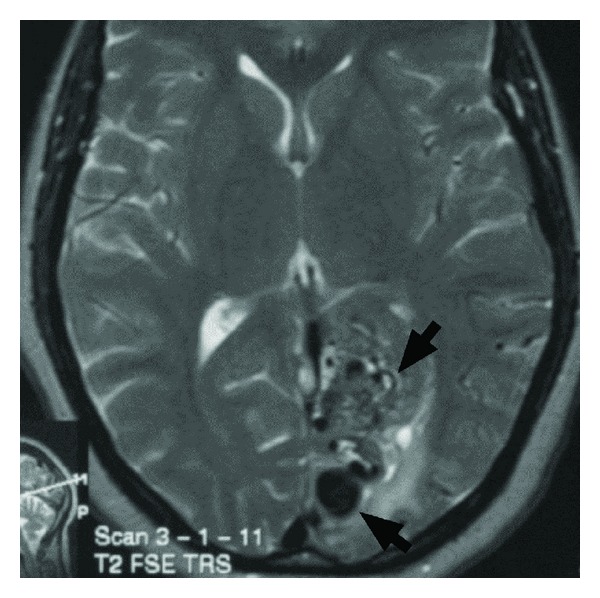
Axial T2 MRI demonstrates a large left parietooccipital arteriovenous malformation yet the patient was found to be a safe driver.
